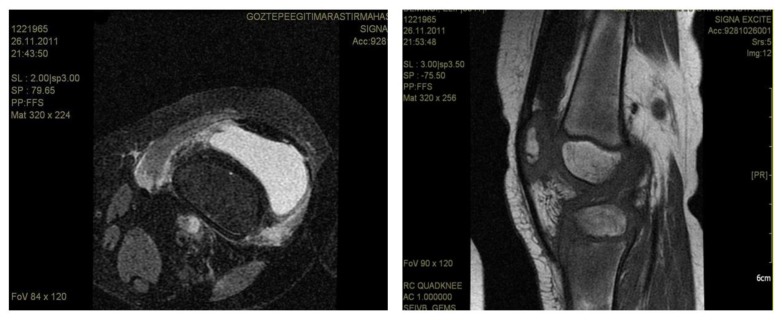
Knee MRI showing synovia inflammation.
